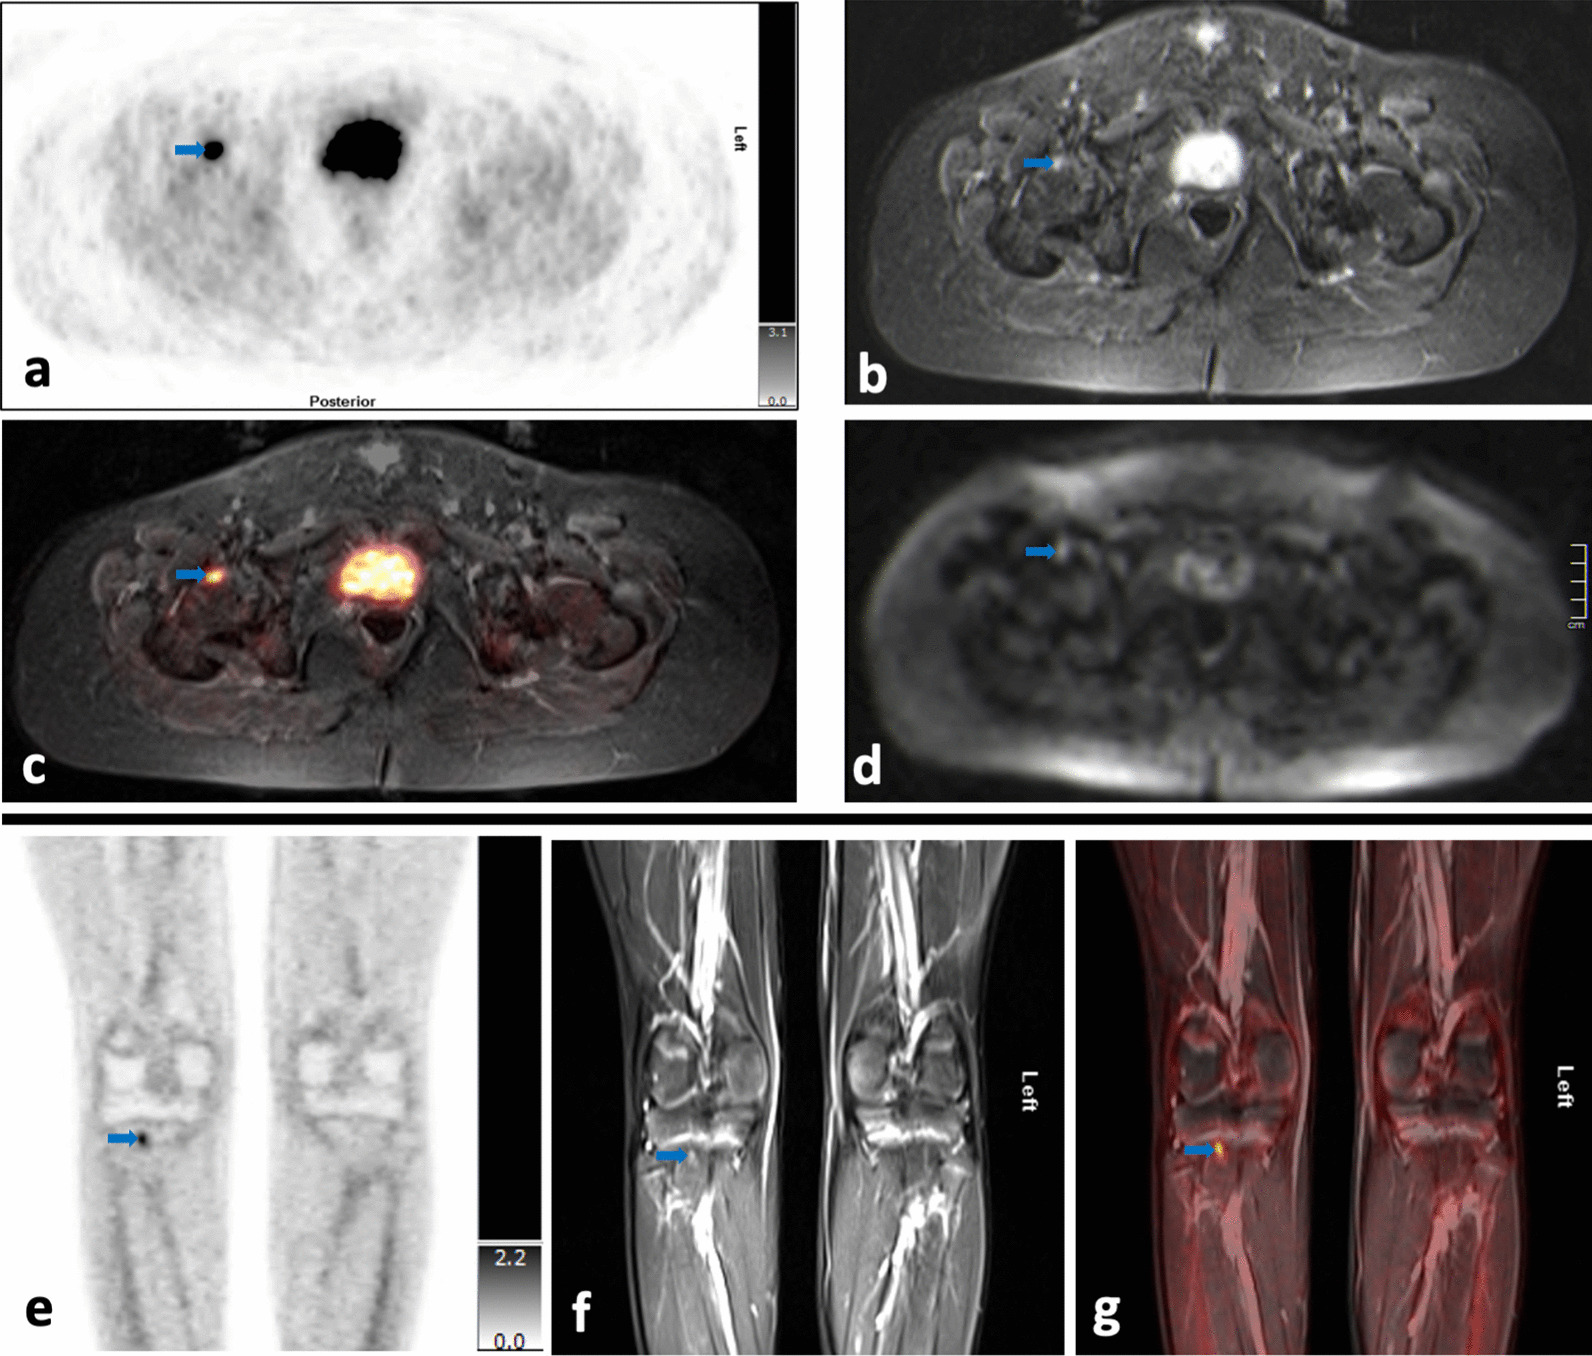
a–g Upper double row: Small focus of skeletal involvement in the right femoral head which has increased glucose metabolism on [^18^F]-FDG-PET images (SUV_max_ 5.98) (a, c), is hyperintense on MR T2-TIRM images (b) and shows restricted diffusion on DWI (d). Lower row: Small focus of skeletal involvement in the right proximal tibia which has moderately increased glucose metabolism on [^18^F]-FDG-PET images (SUV_max_ 2.18) (e, g) and is slightly hyperintense on MR T2-TIRM images (f)
